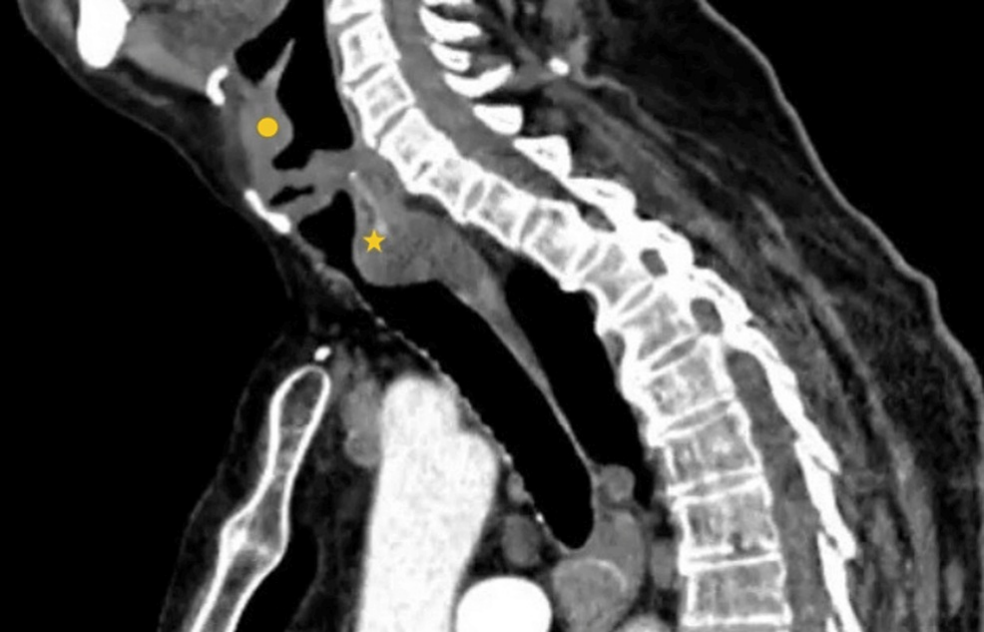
Sagittal CT scan Both masses protrude into the airway, one from the base of the epiglottis on the laryngeal surface, and the other from the posterior part of the subglottic region ⚫: supraglottic mass; ★: subglottic mass
